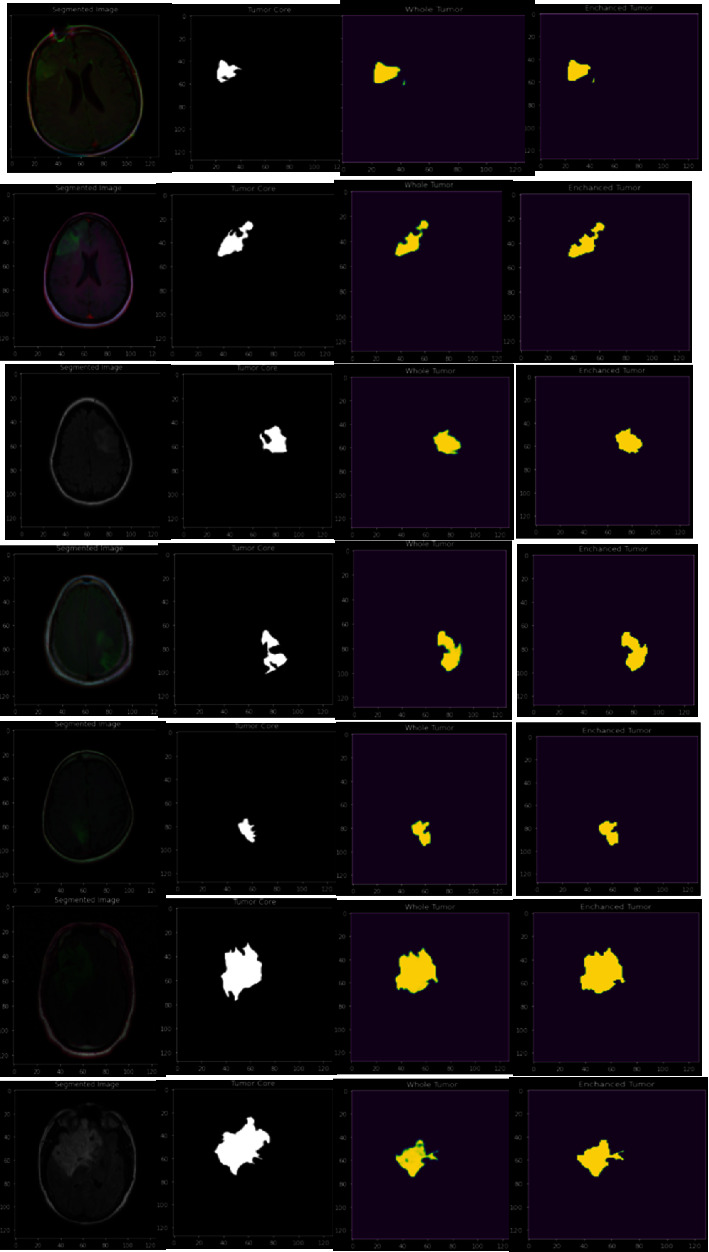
Segmented MRI images as the outcome of the proposed model.
